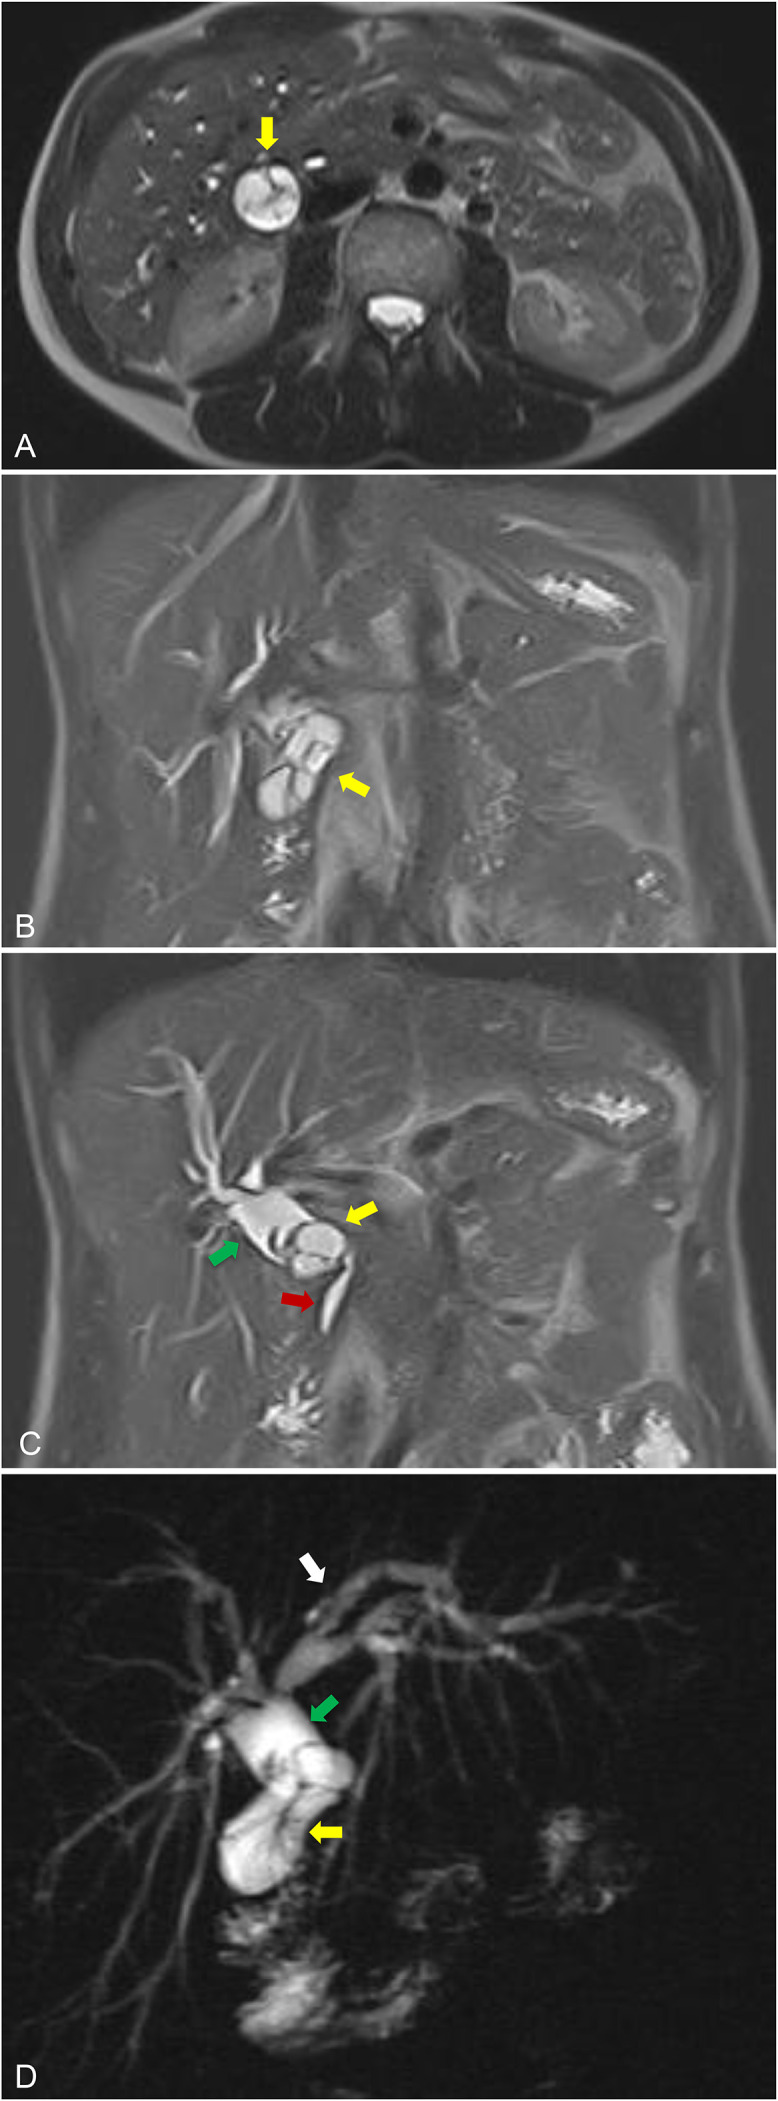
MRI/magnetic resonance cholangiopancreatography (MRCP) of the abdomen: (A) Transverse half Fourier single shot turbo spine echo MR image demonstrates a cystic lesion arising from the gallbladder with internal septations (yellow arrow). (B and C) Coronal half Fourier single shot turbo spine echo MR images demonstrate multiseptated gallbladder lesion (yellow arrows) and associated intrahepatic biliary ductal dilation (white arrow) and common hepatic ductal dilatation (green arrow). There is no significant common bile duct dilatation (red arrow). (D) Heavily T2 weighted thick slab coronal MRCP image demonstrates a multiseptated gallbladder lesion (yellow arrow) as well as intrahepatic (white arrow) biliary ductal and common hepatic (green arrow) ductal dilation.
